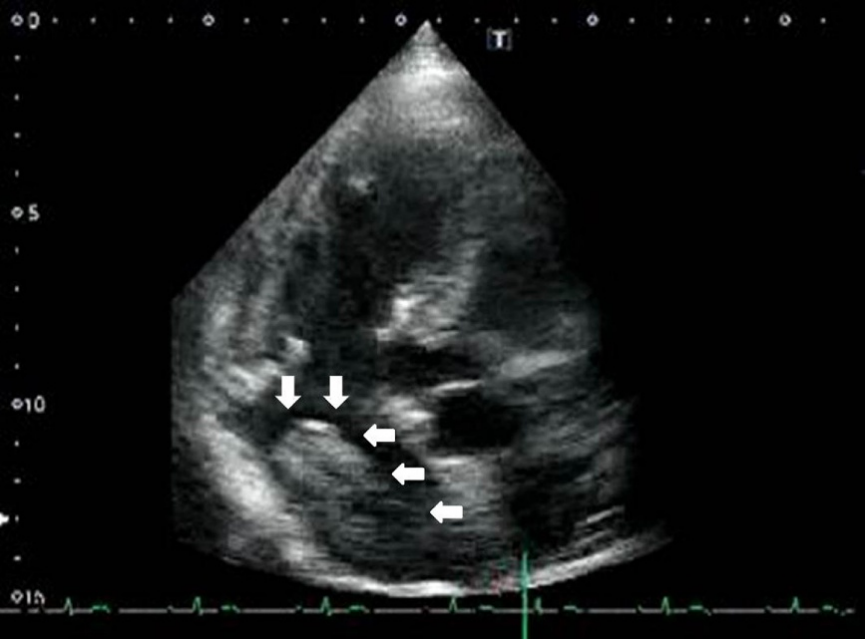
Transthoracic echocardiography shows a 42 × 24 mm left atrium mass (indicated by arrowheads), moving without extension into the outflow tract.
